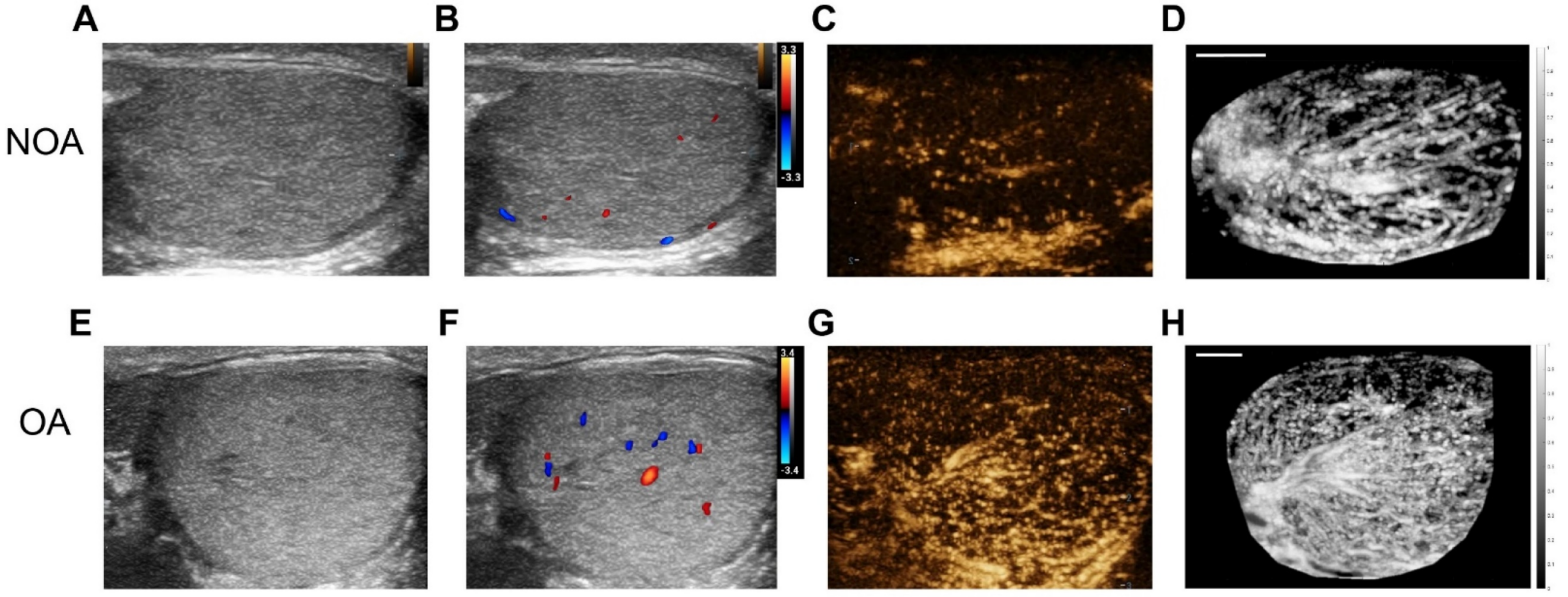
Comparative ultrasonographic assessment of human testes in azoospermia patients. The top row shows images from a patient with non-obstructive azoospermia (NOA), and the bottom row depicts images from a patient with obstructive azoospermia (OA). From left to right: (A) & (E) B-mode ultrasound, (B) & (F) color Doppler flow imaging (CDFI), (C) & (G) contrast-enhanced ultrasound (CEUS), and (D) & (H) maximum intensity projection (MIP) images. To facilitate an intuitive comparison, the NOA images were horizontally mirrored. Scale bars = 5 mm.
